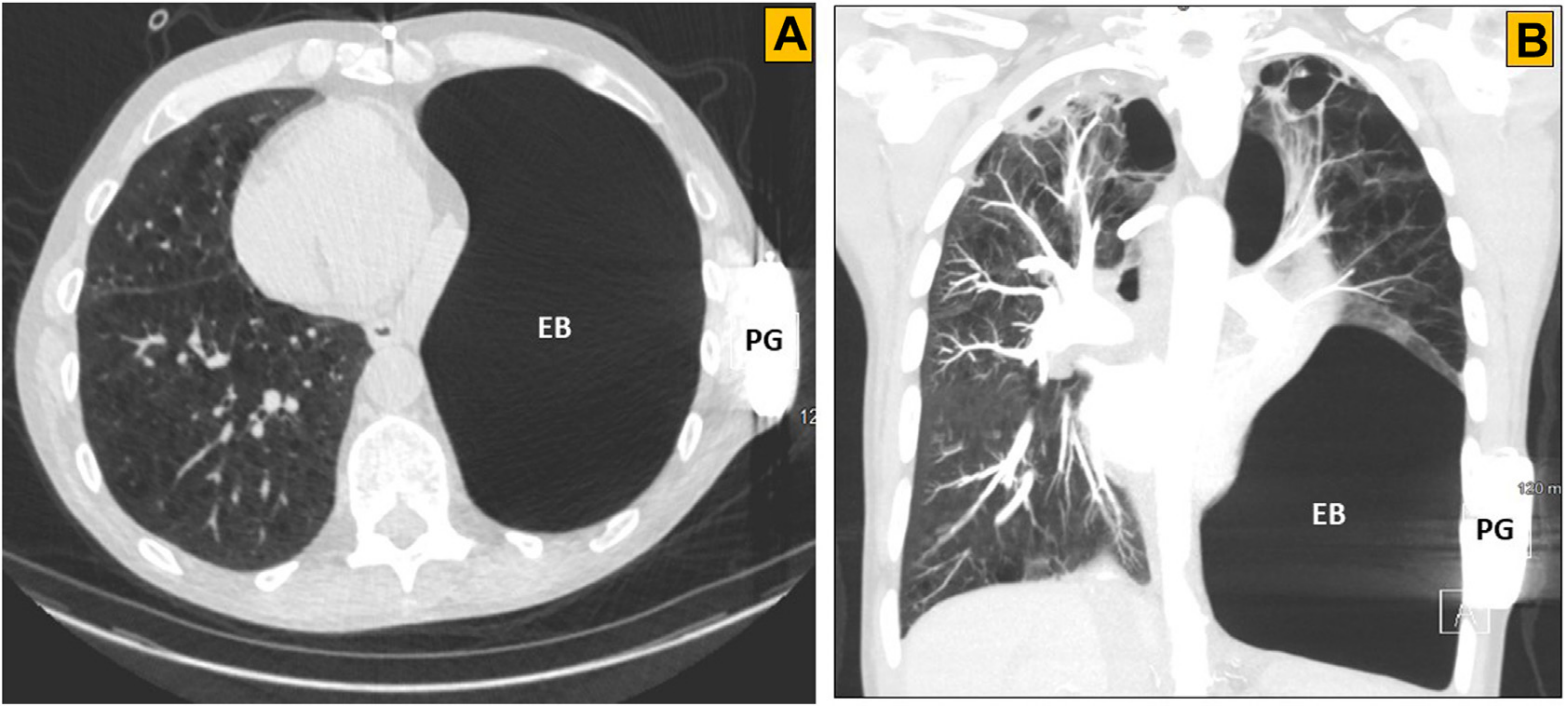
Computed tomography scan of the chest showing advanced emphysema, traction bronchiectasis, and a large emphysematous bulla occupying entire left lower hemithorax in shock path. A: Coronal view. B: Sagittal view. EB = emphysematous bulla; PG = pulse generator.
